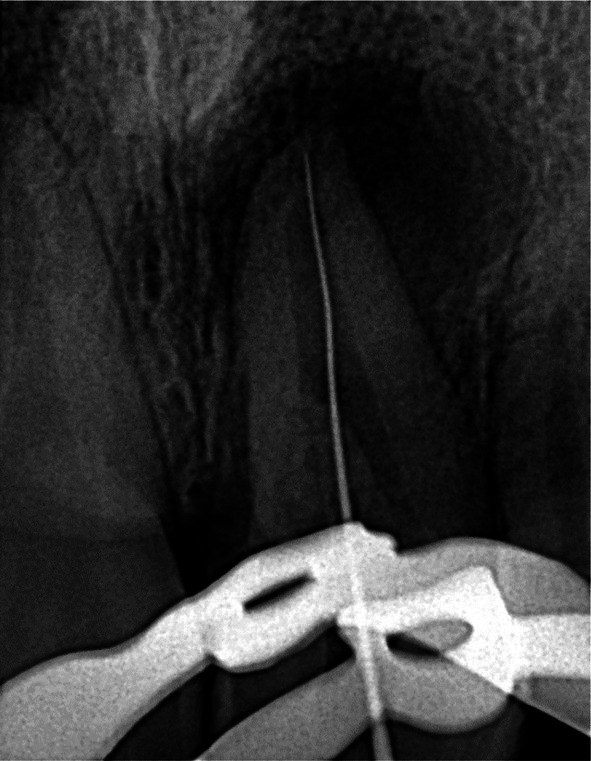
Initial file radiography.
